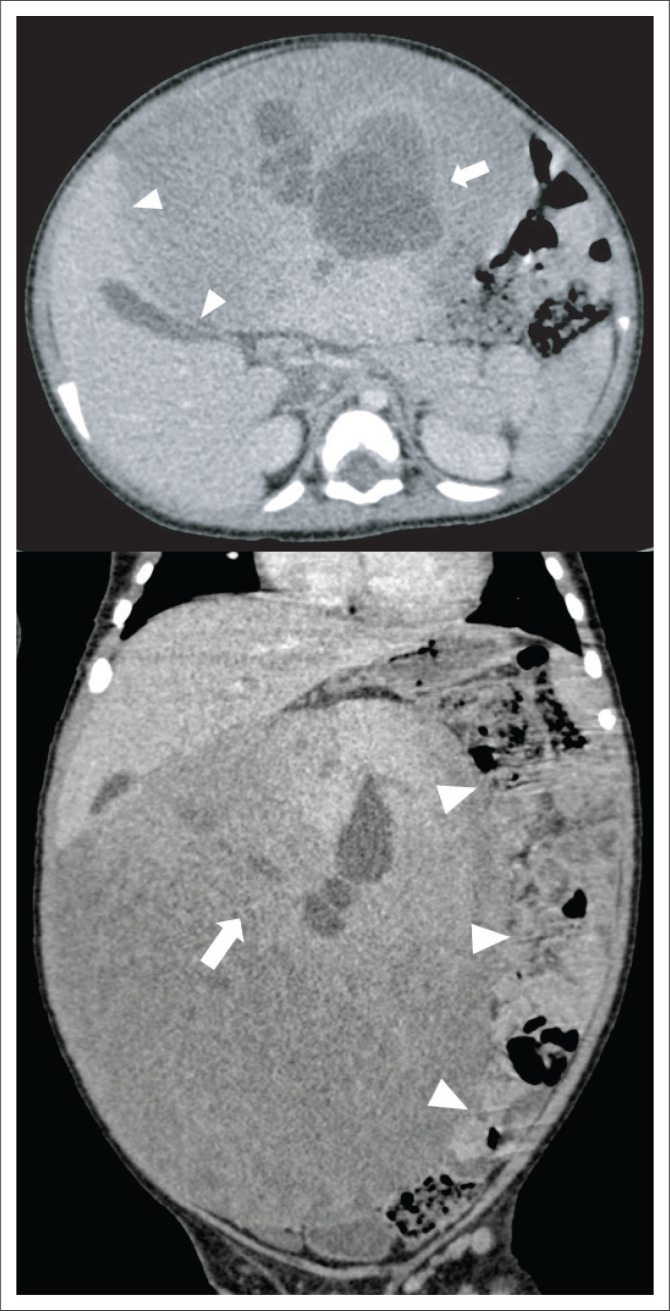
Initial imaging – Axial and coronal contrast-enhanced computed tomography of the abdomen (portal venous phase) demonstrates a large, complex mass lesion in the right abdomen. It abuts the inferior liver border with a poor plane of separation and displaces the bowel to the left (arrowheads). There is a lack of peripheral hepatic parenchymal extension along the edges of the mass (claw sign); therefore, hepatic origin cannot confidently be suggested. Solid and cystic elements are clearly distinguishable (arrow) with the classic ‘swiss cheese appearance’.
